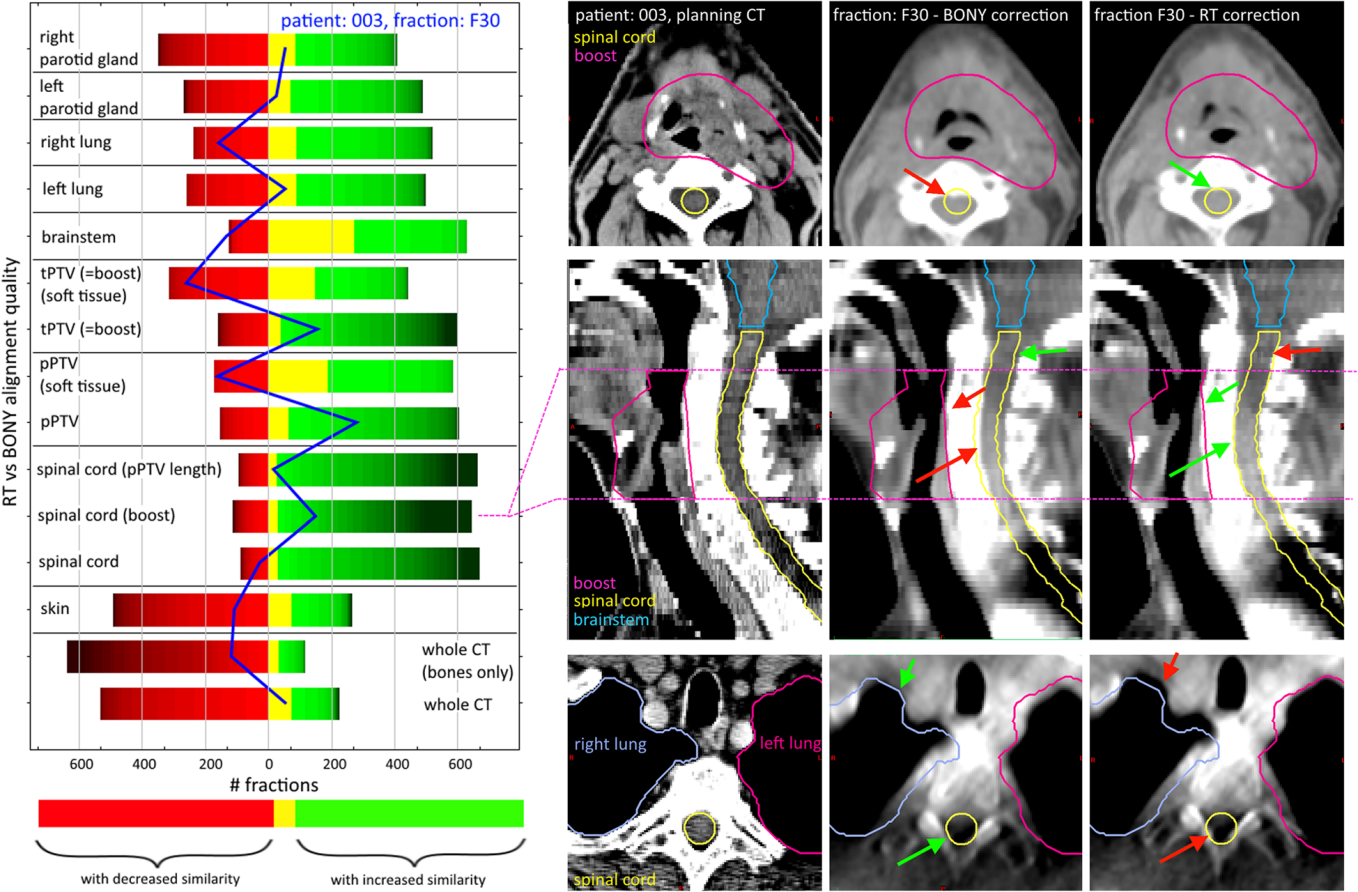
Left panel:* Histogram displaying VOIs that increase (*green*) or decrease (*red*) their local similarity by the manual expert-correction in respect to the bony pre-match. The *blue line*, belonging to one exemplary fraction and patient, reflects the trade-off in the VOI alignment process. While for this selected patient geometry the structure alignment for spinal cord (boost) was improved, the whole spinal cord structure experienced a degradation of alignment. *Right panel*: CT views of the selected patient 003 visualizing the trade-off in the alignment quality of local structures. The VOIs indicate corresponding positions in the planning situation. The *dotted line* indicates the cc extension of the spinal cord (boost) substructure. The *green arrows* mark location with visible improvement of the alignment quality of the fraction scan in regard to the planning CT. The *red arrows* are the counterpart of the green ones, marking a decline of alignment quality
